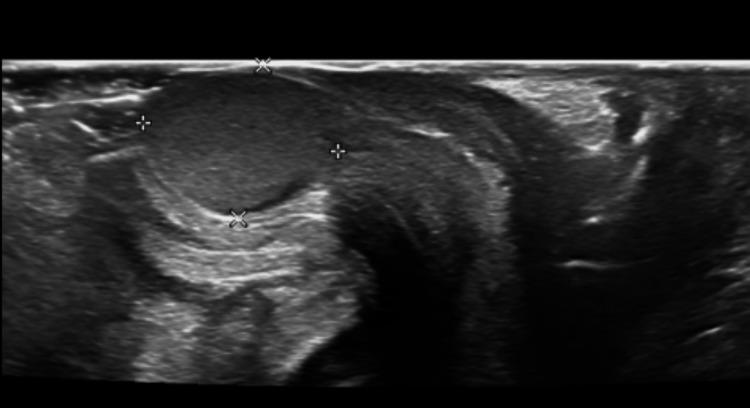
Sagittal translabial ultrasound view Sagittal translabial view showing the 1.1 x 1.0 x 1.0 cm mass in the vaginal canal. It is homogeneous and isoechoic. There is mild posterior acoustic enhancement. The presence of echogenicity and absence of an anechoic center made the lesion appear solid rather than cystic, which may have contributed to its misinterpretation as testicular tissue.
